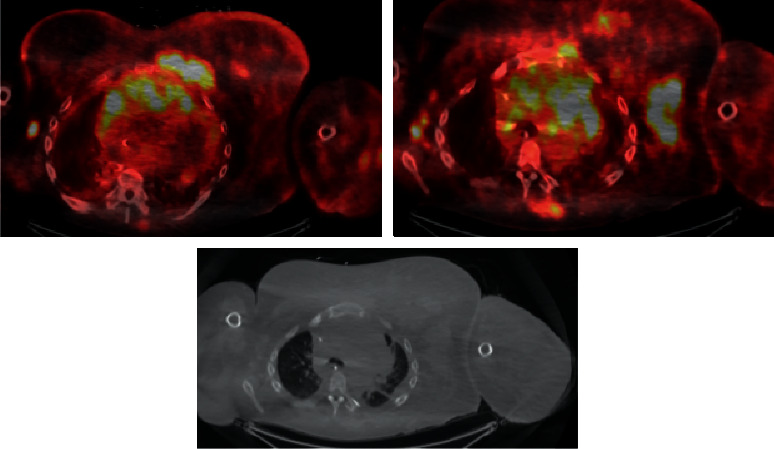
Initial PET/CT showing significant hypermetabolic activity above the diaphragm specifically located in the L axillary, mediastinal, and thoracic wall conglomerate.
